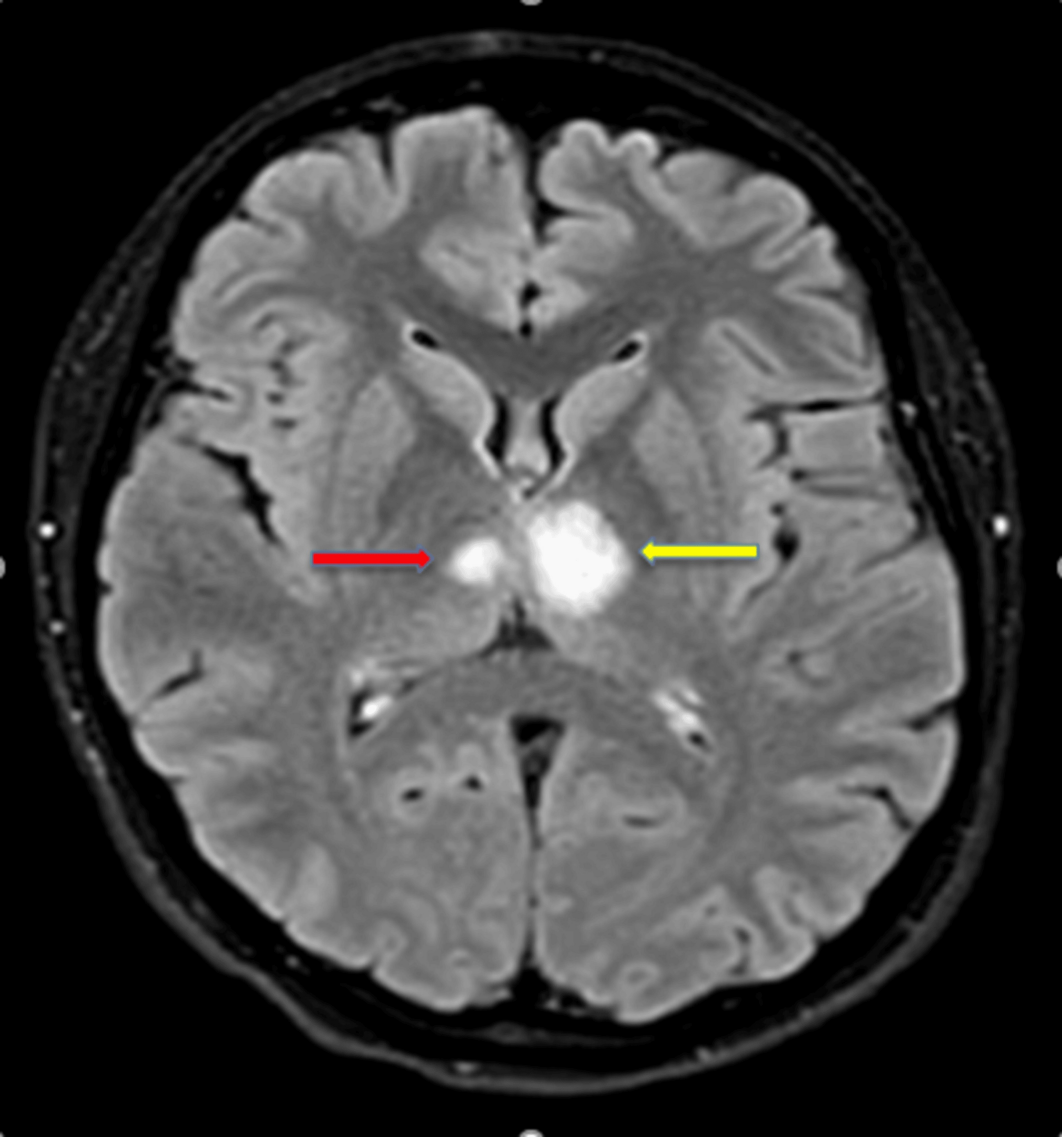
Fluid-attenuated inversion recovery (FLAIR) imaging showing two sizable foci of hyperintense lesions in the bilateral thalami. The lesion on the right measured 12 mm (red arrow), and the lesion on the left measured 22 mm (yellow arrow). These lesions were also noted in T2-weighted imaging and diffusion restriction on diffusion-weighted imaging (DWI). These features are consistent with acute infarcts.
